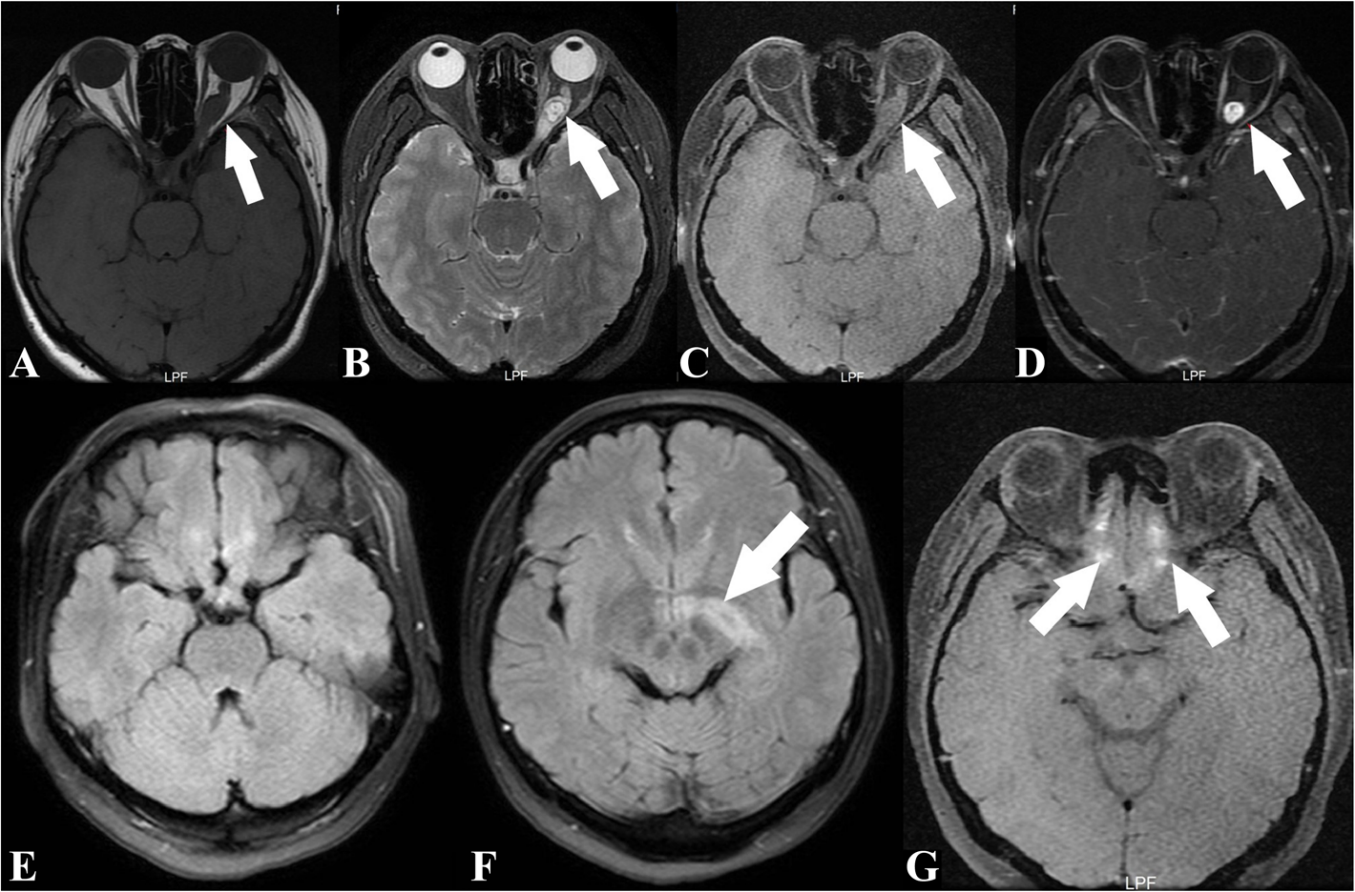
Comparison of preoperative and postoperative MRI. The T1- and T2-weighted images show optic neuropathy before the operation. The T1 image shows isointensity (a), and the T2 and FLAIR images show hyperintensity (b, c). There was a significantly enhanced signal in the tumour after the enhancement scan (d). The FLAIR image shows hyperintensity of the left optic chiasma, visual radiation, and bilateral frontal lobe (e, f, g)
